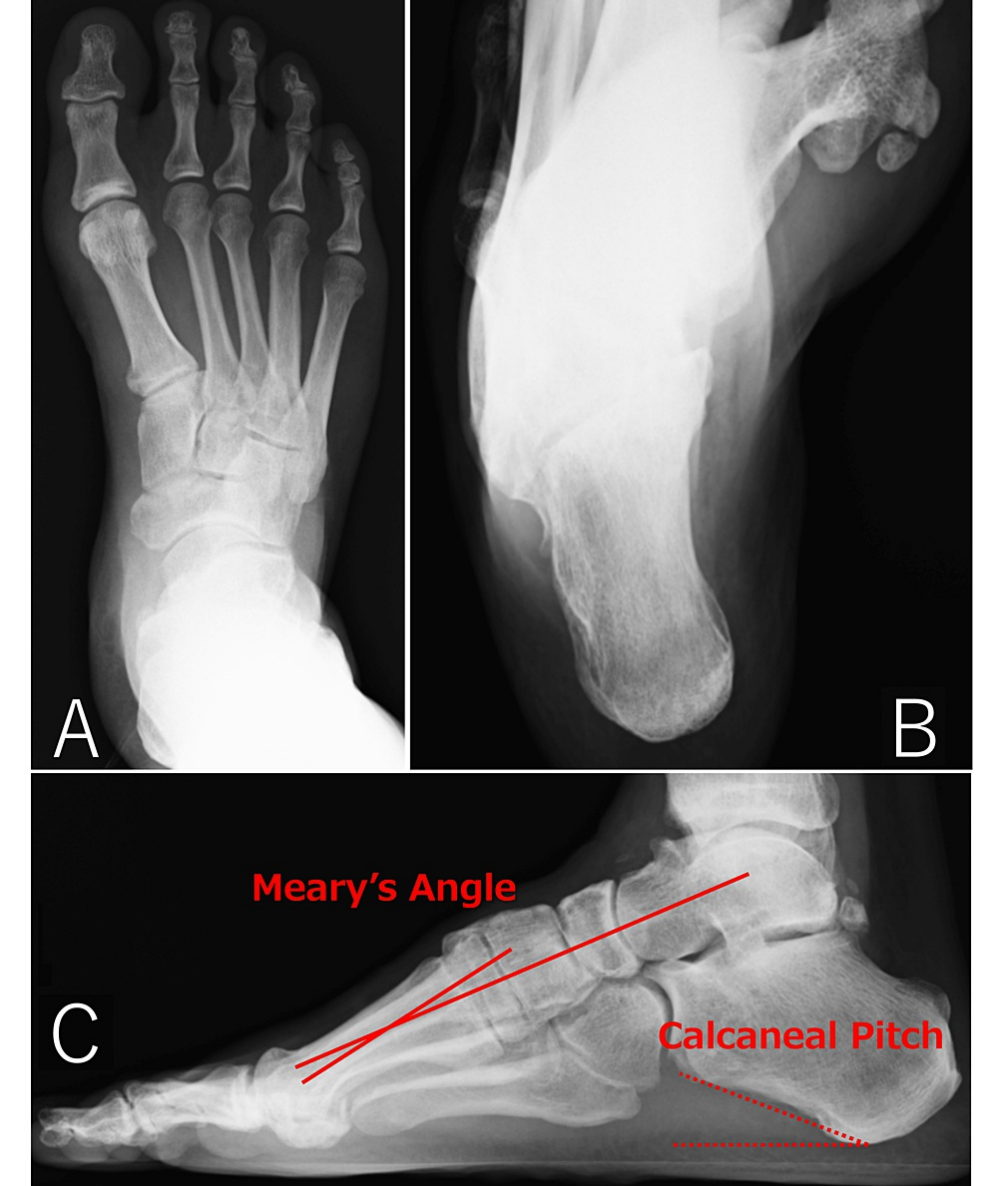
Plain weight-bearing radiographs (A) Dorsoplantar view. The avulsion fracture of the distal fibula and the fracture of the fifth metatarsal, which are the patient's post injuries are not seen in the images. (B) Non-weight bearing axial view of the calcaneus. Marked varus was seen at the right calcaneus. (C) Lateral view. Meary’s angle was 13° upward convex, the calcaneal pitch was 23°, and the navicular bone was elevated markedly above the floor.
